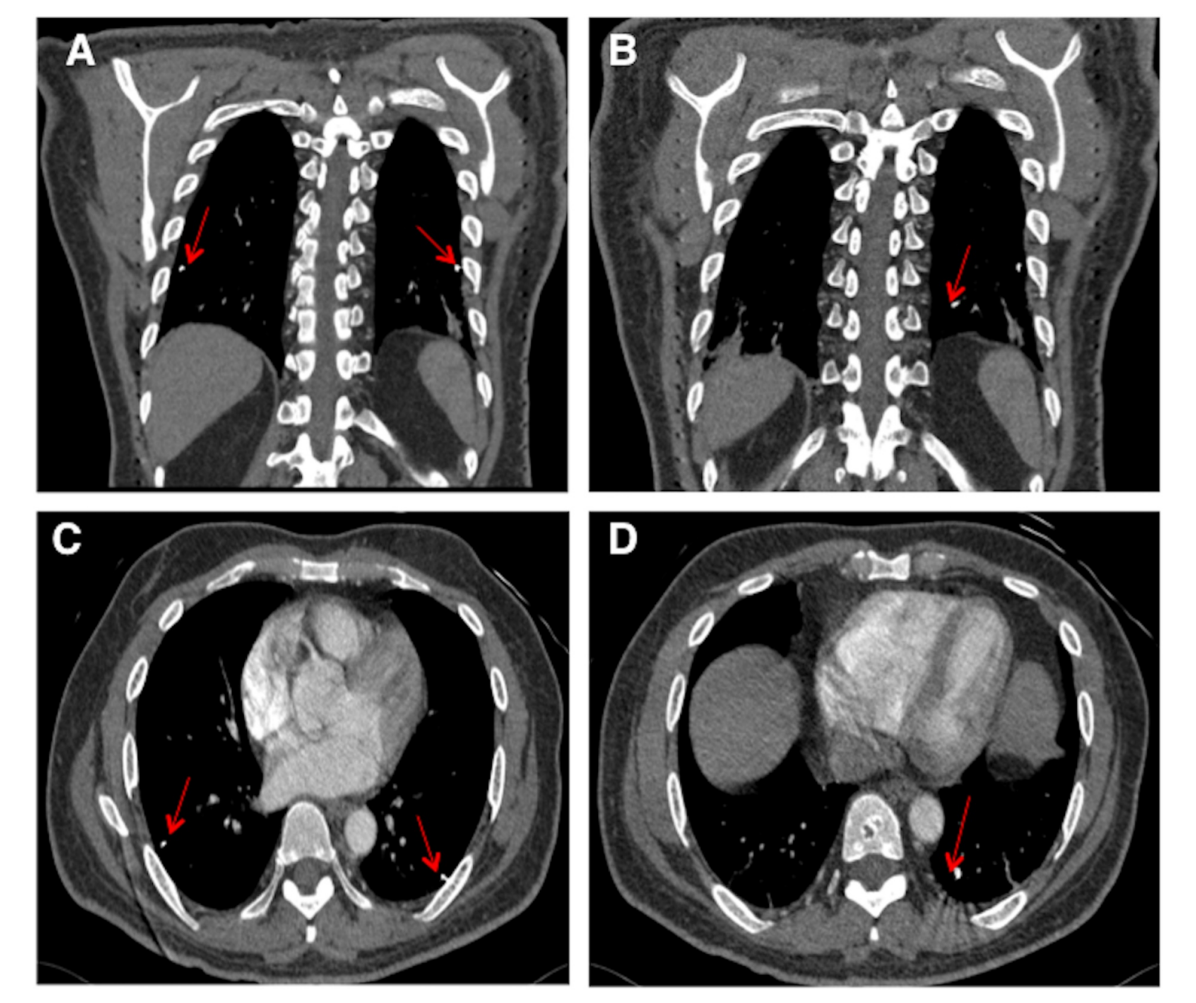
Coronal (A and B) and axial (C and D) CT of the chest with contrast showing the three brachytherapy seeds, which were visualized on chest radiograph.
